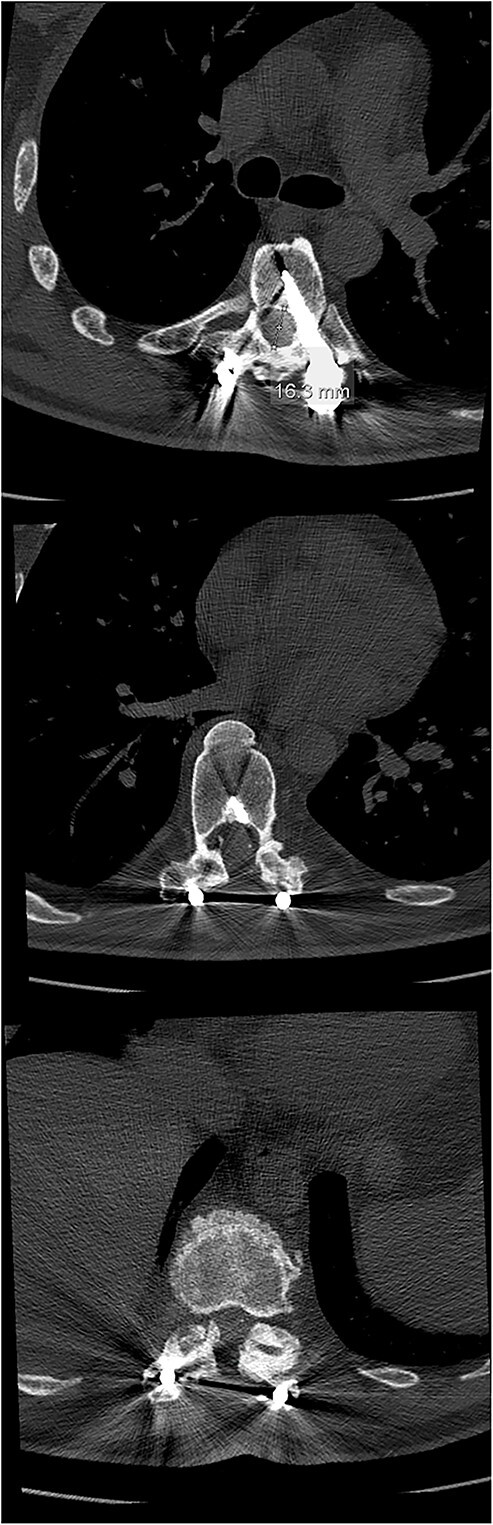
Final follow-up CT scan (axial views), showing adequate width of the spinal canal at Th6 (above), Th9 (middle) and Th11 (below).
